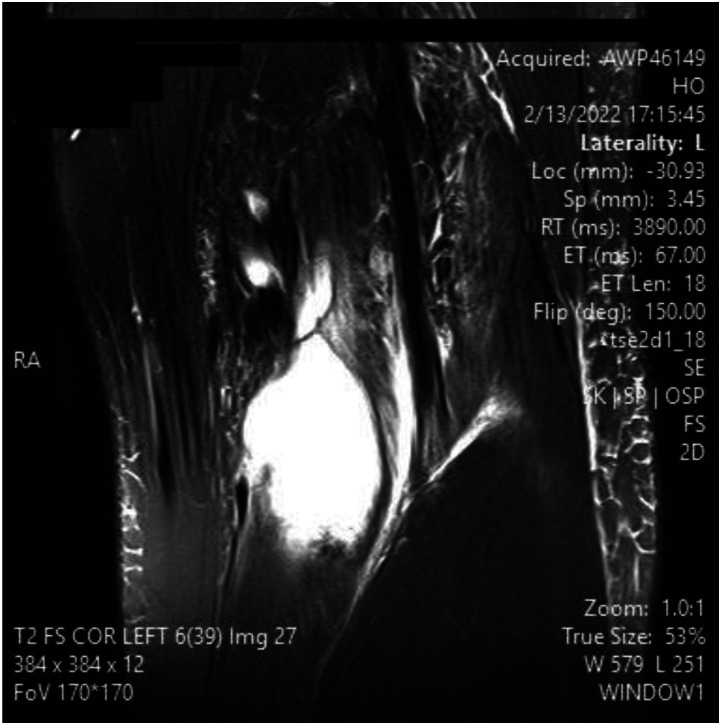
MRI slices on T2 signal showing increased signal of Left knee popliteal bursa. Figure 4 shows a coronal slice
